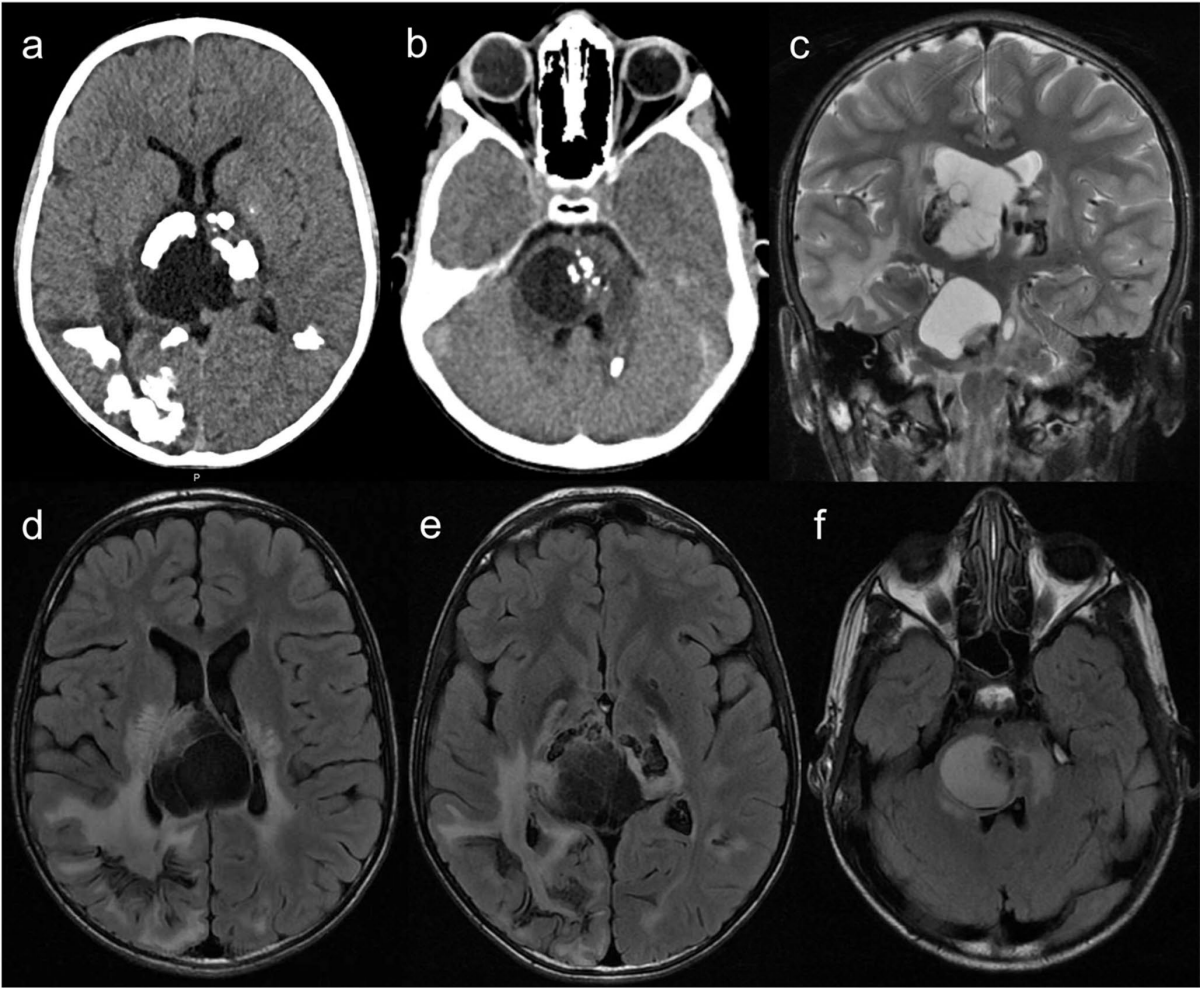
Cerebroretinal microangiopathy with calcifications and cysts. A 13-year-old male patient with asymmetric spastic tetraparesis, bilateral dysmetria, and right-sided amaurosis. Head CT scan (a and b) and brain MRI (c – coronal T2, and d to f – axial FLAIR) reveal coarse calcifications in the subcortical white matter, basal nuclei, and cerebellum. Intra-axial cysts are observed in the pons and right thalamus, with progressive growth (previous scans not shown), compressing the adjacent ventricular system but without hydrocephalus; the pontine cyst located exhibits higher signal than CSF on FLAIR, reflecting high protein content. There is also extensive T2 hyperintensity in the white matter, particularly around the cysts
